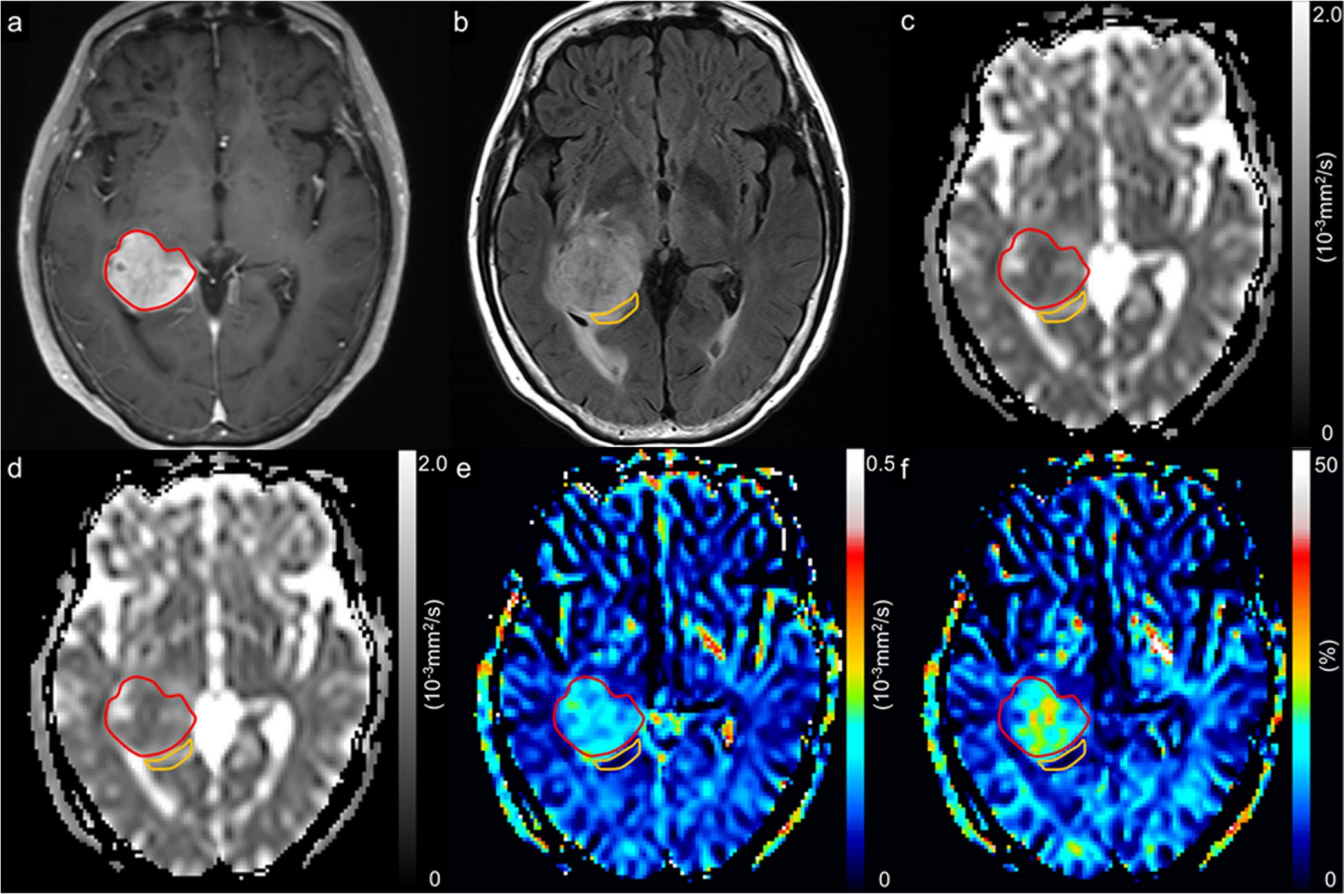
A 74-year-old woman with glioblastoma, isocitrate dehydrogenase-wildtype, grade 4. A contrast-enhanced T1-weighted image with a region of interest of the enhancing region (red line) (a), a FLAIR image with a region of interest of peritumoral region (orange line) (b), an apparent diffusion coefficient (ADC) map derived from pulsed gradient spin-echo (PGSE) DWI at an effective diffusion time (Δ_eff_) of 44.5 ms (c), an ADC map derived from oscillating gradient spin-echo (OGSE) DWI at an Δ_eff_ of 7.1 ms (d), and maps of ADC change between PGSE DWI and OGSE DWI (cADC) (e) and relative ADC change between PGSE DWI and OGSE DWI (rcADC) (f). The ADC values in the tumor appear higher at short Δ_eff_ values than at long Δ_eff_ setting. Small changes in cADC and rcADC are noted between the OGSE and PGSE sequences in the tumor
